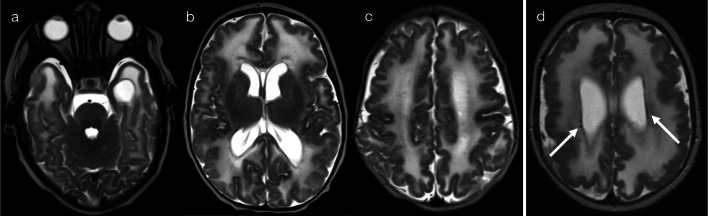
T2 hyperintensity in supratentorial white matter—with neuronal migration abnormality (Pattern 4A). Case 1: 11-week-old infant with bilateral sensorineural hearing loss on newborn testing. Guthrie test was positive for CMV. T2-weighted imaging (a–c) demonstrated polymicrogyria with diffuse white matter hyperintensity and cystic change in the left anterior temporal pole. Clinical notes at 14 months of age indicated developmental delay. Case 2: 4-month-old infant with microcephaly, developmental delay and hypertonia under investigation. Guthrie test was positive for CMV. T2-weighted imaging (d) demonstrated polymicrogyria with diffuse white matter hyperintensity and subtle periventricular calcifications (arrows)
